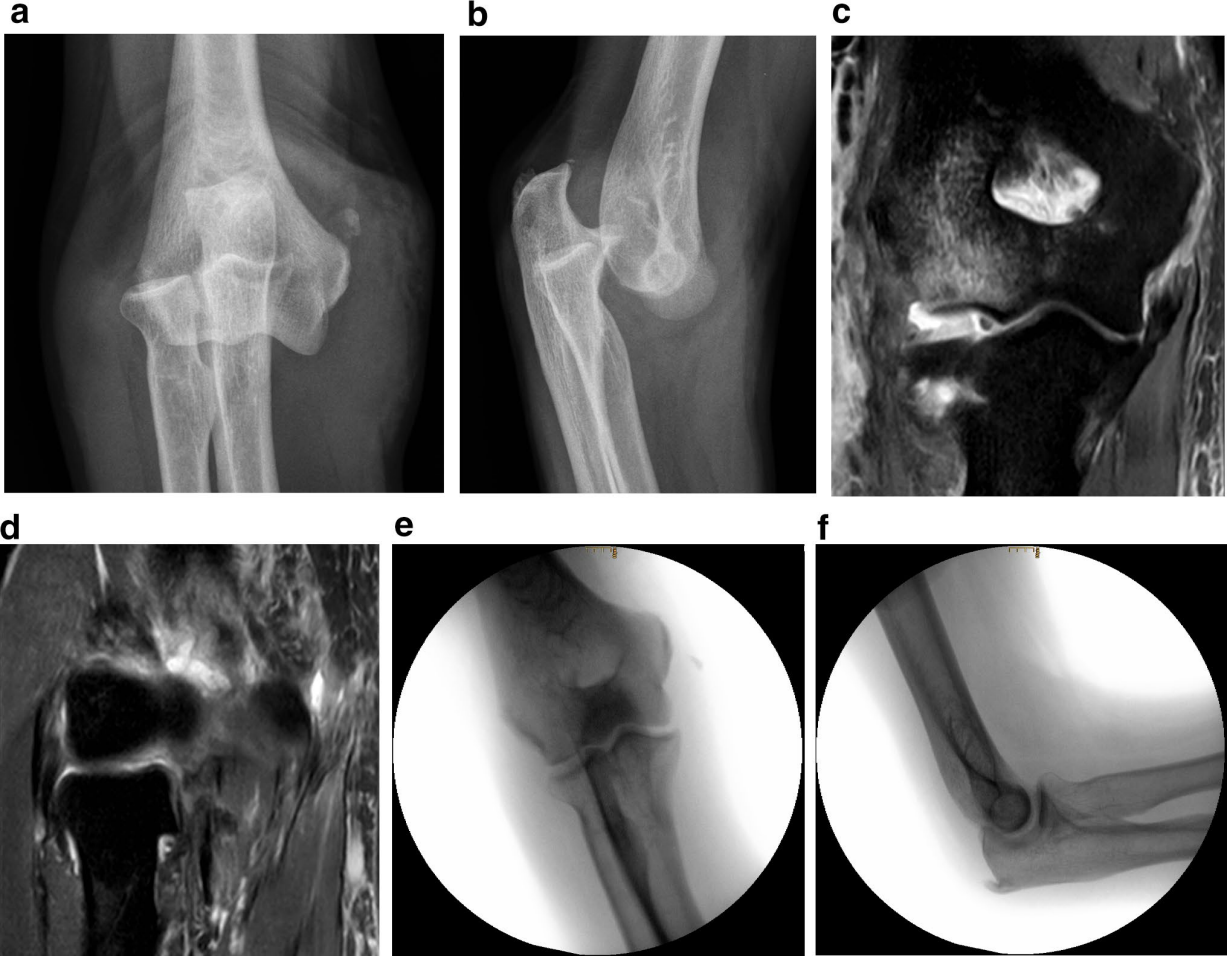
a, b Anteroposterior and lateral radiograph of a posterior elbow dislocation of a 51-year old patient after simple fall from standing height. c Coronal short tau inversion recovery (STIR) sequence shows signal abnormalities of the MCL and CFO (complete tear) as well as severe bone marrow edema laterally. d The LCLC is partially detached from its humeral origin, whereas CEO are intact. The functional integrity of the LCLC and CEO is confirmed by stress test under dynamic fluoroscopy: e no joint gapping during varus stress and f centered joint in the lateral projection under fluoroscopy
